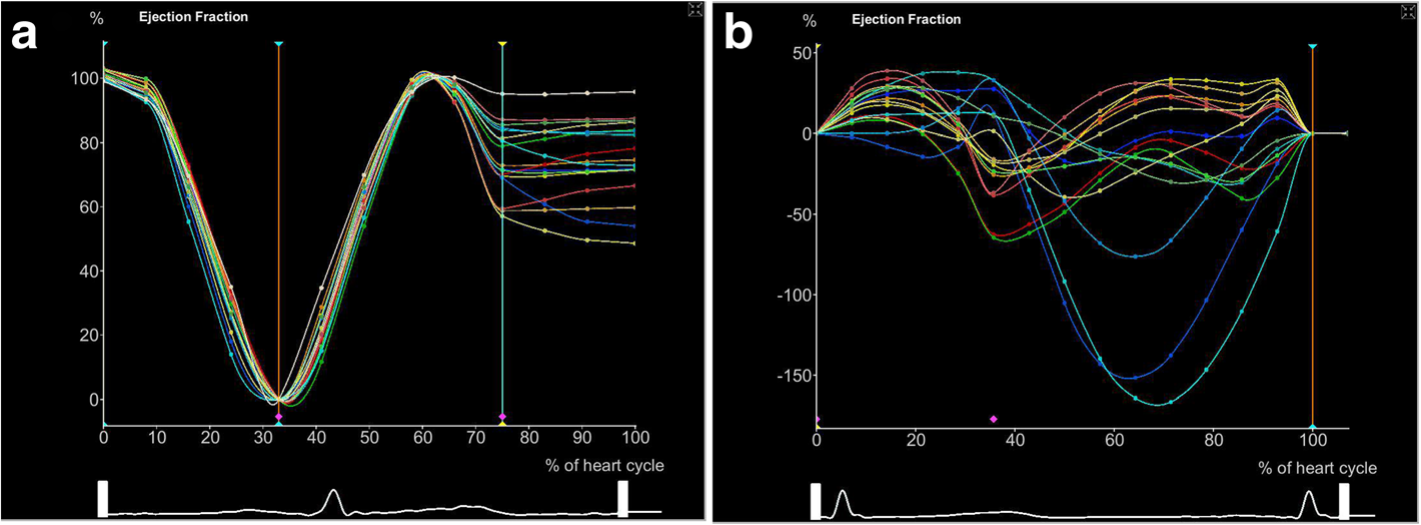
Segmental ejection fraction (EF) (%) of healthy controls (a) and patient cases (b) by real-time three-dimensional echocardiography (RT-3DE)
